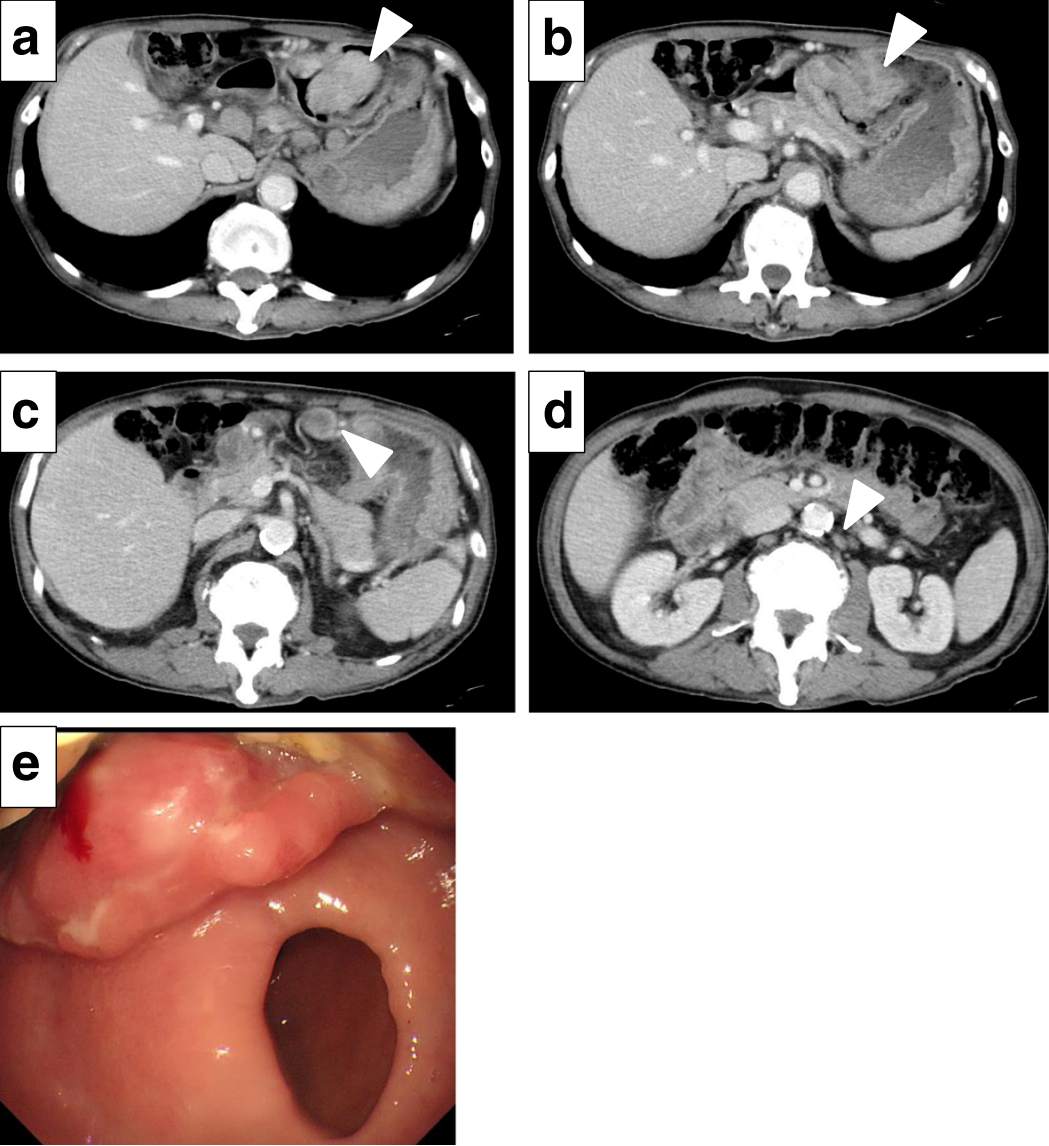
Examination images of the gastric cancer before the operation. a Abdominal computed tomography revealing an enhancing mass in the gastric antrum (*white arrow head*). b A more distal section of the gastric tumor with evidence of pyloristenosis (*white arrow head*). c Peri-gastric lymph node enlargement (*white arrow head*). d Enlarged para-aortic lymph nodes (*white arrow head*). e Esophagogastroduodenoscopy revealing type 2 gastric cancer approaching the pylorus
